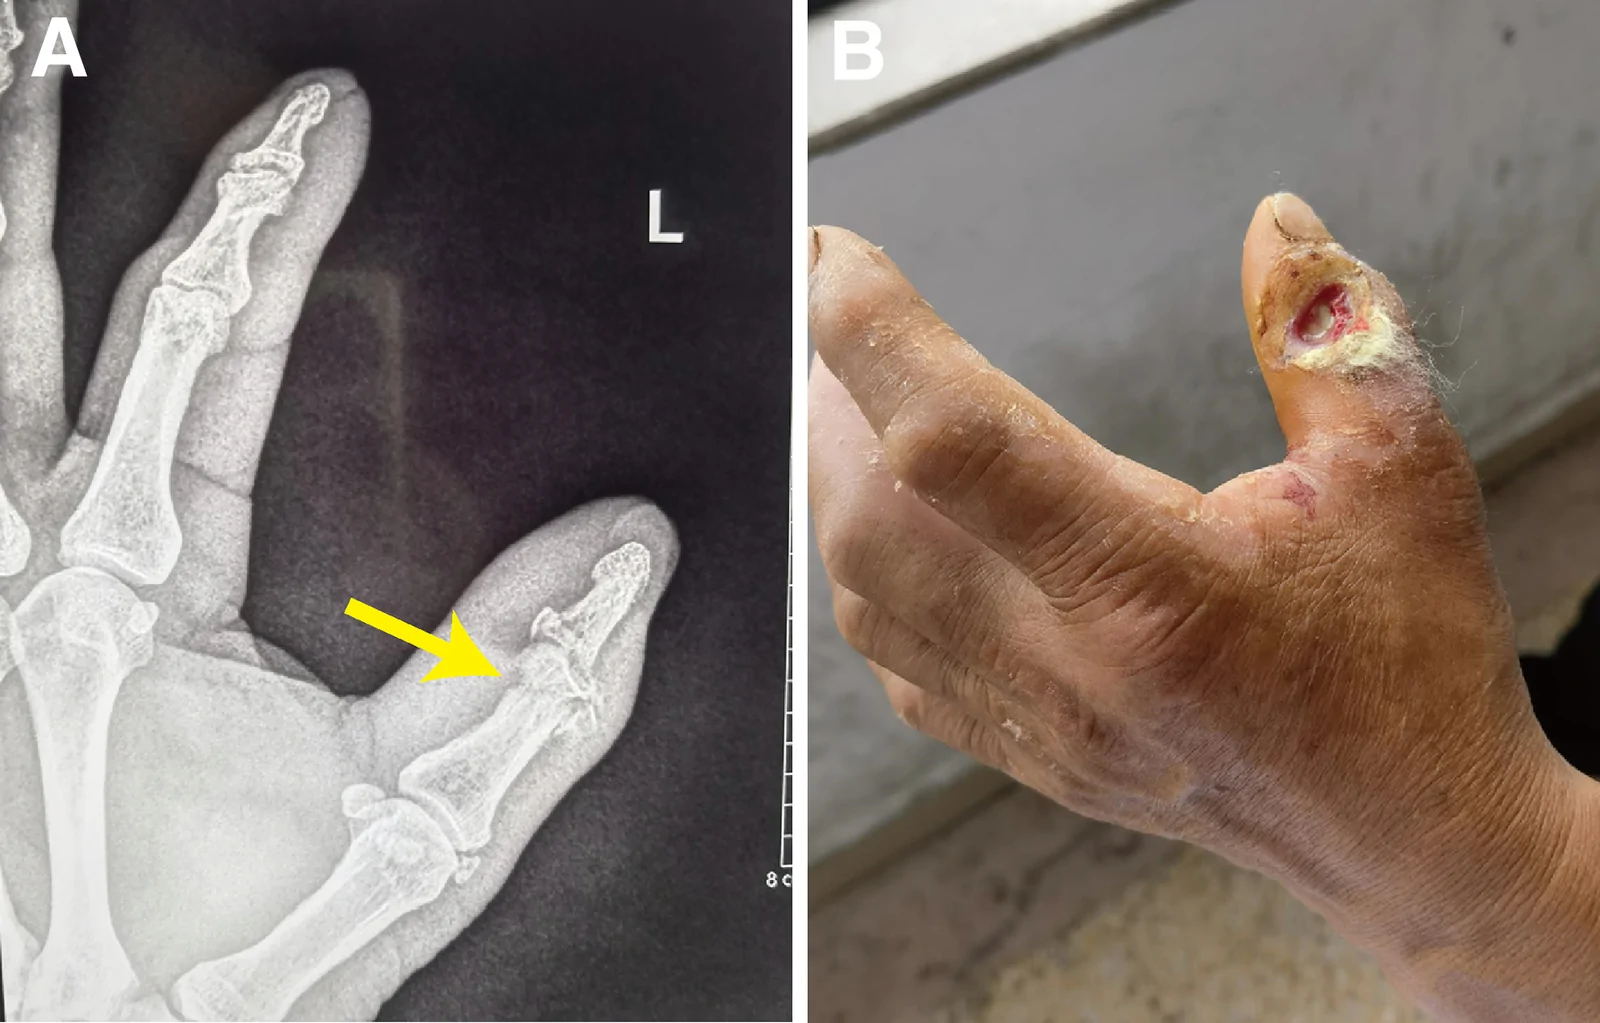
(A) X-ray showing a comminuted fracture of the left thumb phalanx (yellow arrow). (B) Skin ulcer on the medial side of the thumb.
